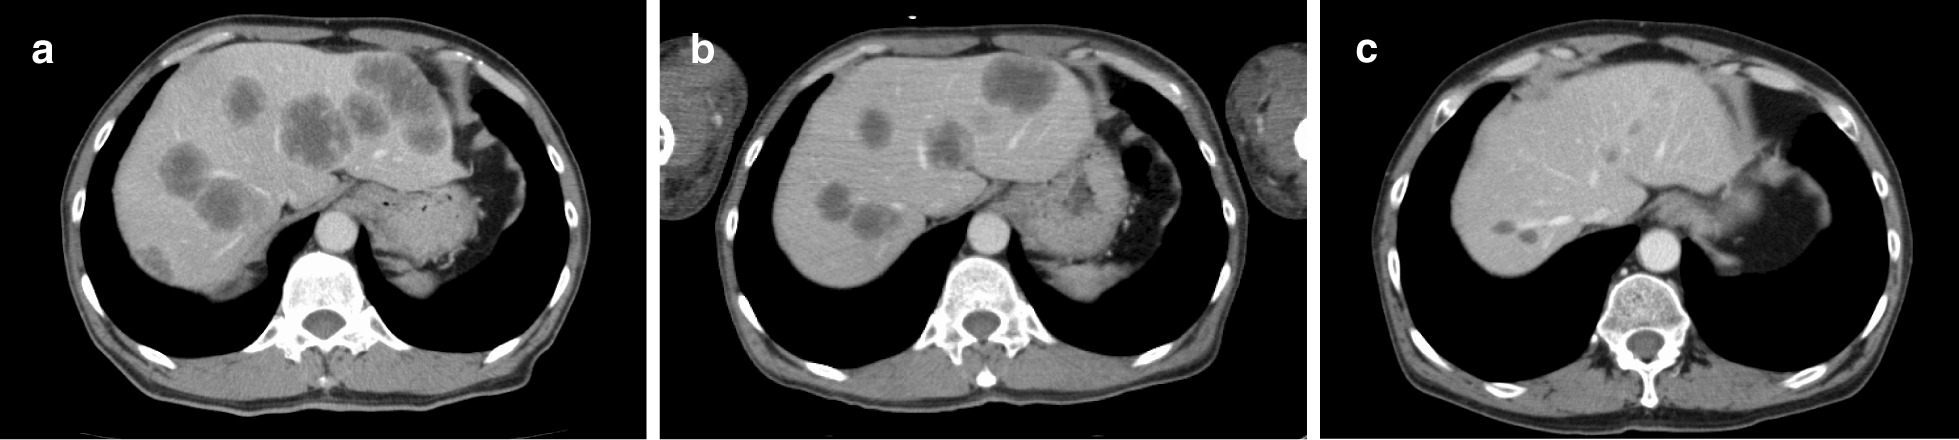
CT findings of liver metastases before ADT (a), before EP therapy (b), and after 4 courses of EP therapy (c)
